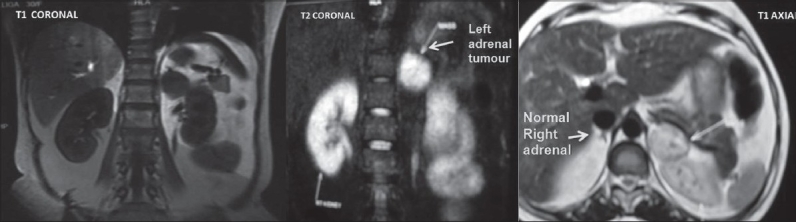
Magnetic resonance imaging of the abdomen-T1 and T2 weighted images showing a 3.5×3 cm, mixed intense mass in the left adrenal gland. Contralateral adrenal gland was normal
